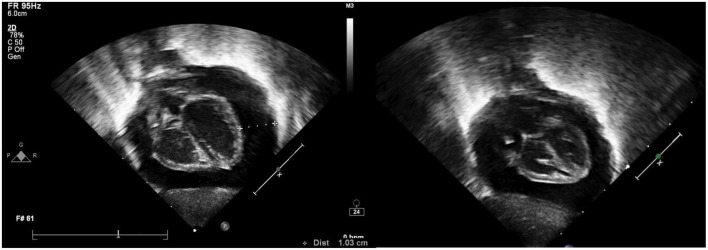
Echocardiogram showing cardiac tamponade of Case III.
